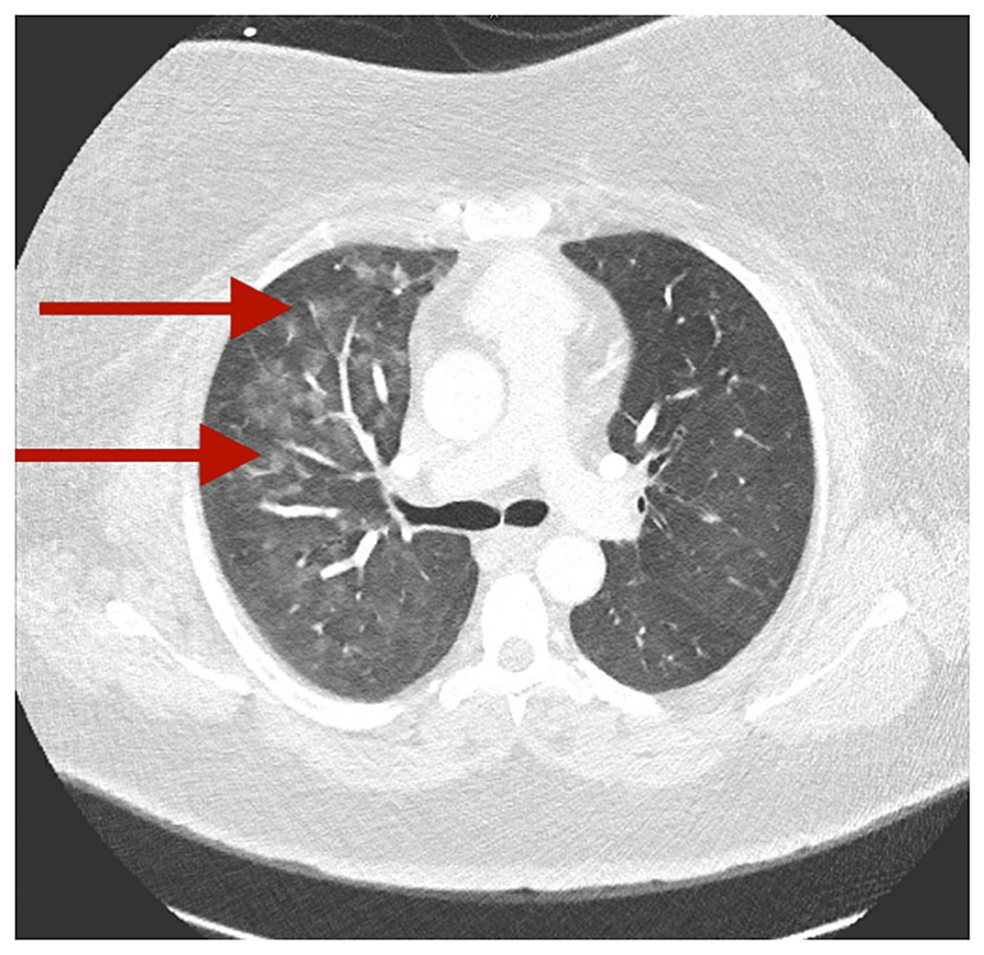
CT chest with contrast (diffuse fluffy ground glass opacities bilaterally involving the right upper and middle lobes)
